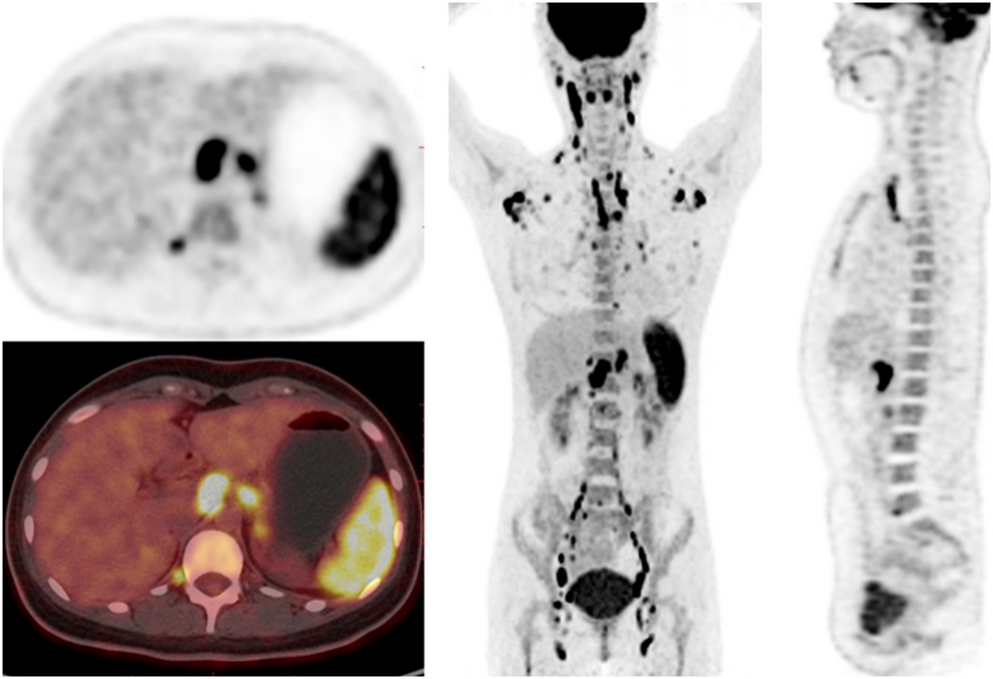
^18^F-FDG PET/CT images in a 23-year-old female of AOSD patient. PET/CT showed diffusely increased ^18^F-FDG uptake in spleen, bone marrow and lymph nodes, and reactive hyperplastic lymph nodes symmetrically distributed in the whole body.
